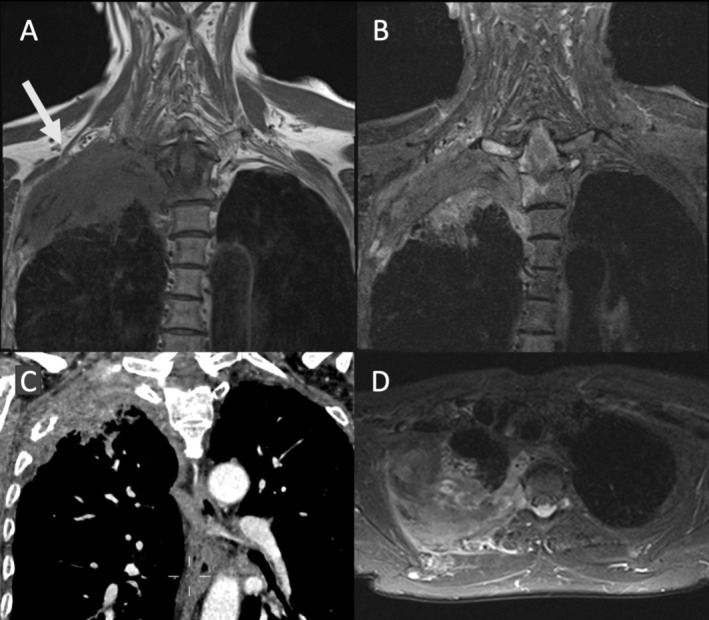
Superior sulcus (Pancoast) tumor in a 62‐year‐old female patient (arrow). Coronal T1‐weighted fast spin echo (A) and STIR images (B) and the axial STIR image (C) clearly show tumor invasion from the right lung apex into the thoracic wall and vertebral column with identification of the brachial plexus and superior soft tissue contrast compared to CT (C).
